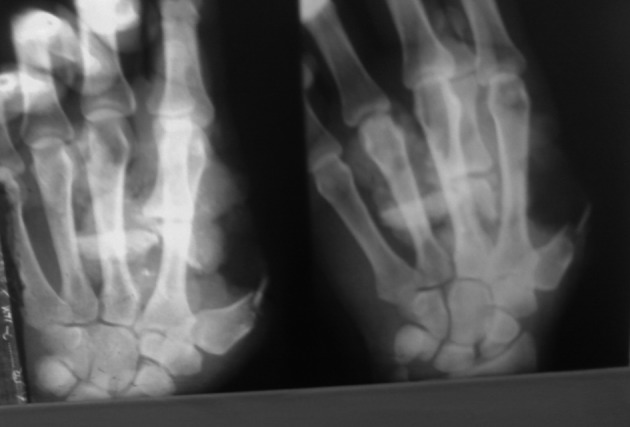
Radiography of Patient's Hand Reveals an Incompletely Amputated Thumb of the Left Hand
